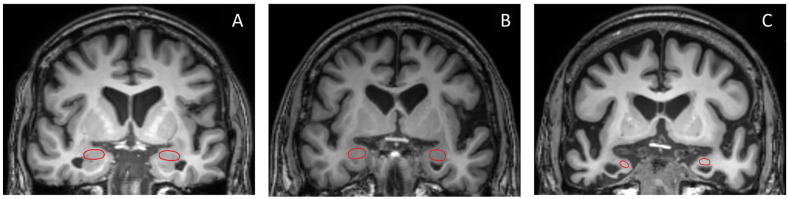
Coronal T1W brain MR images of (A) NC, (B) MCI, and (C) dementia subjects are presented in this study. The red circle highlights the amygdala.
